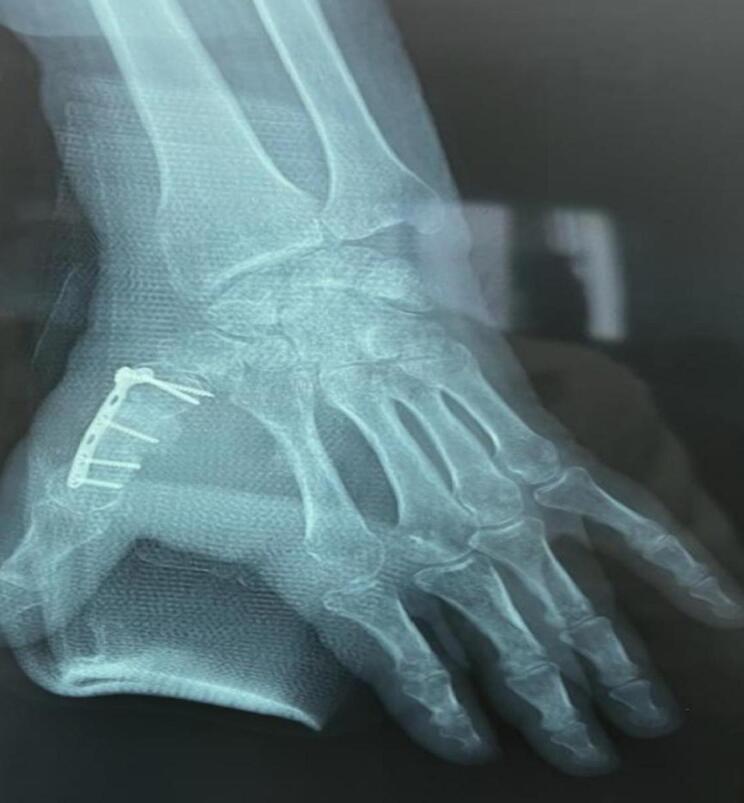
X-ray at the 6-week follow-up of the second stage of the Masquelet procedure, showing good bone consolidation.
